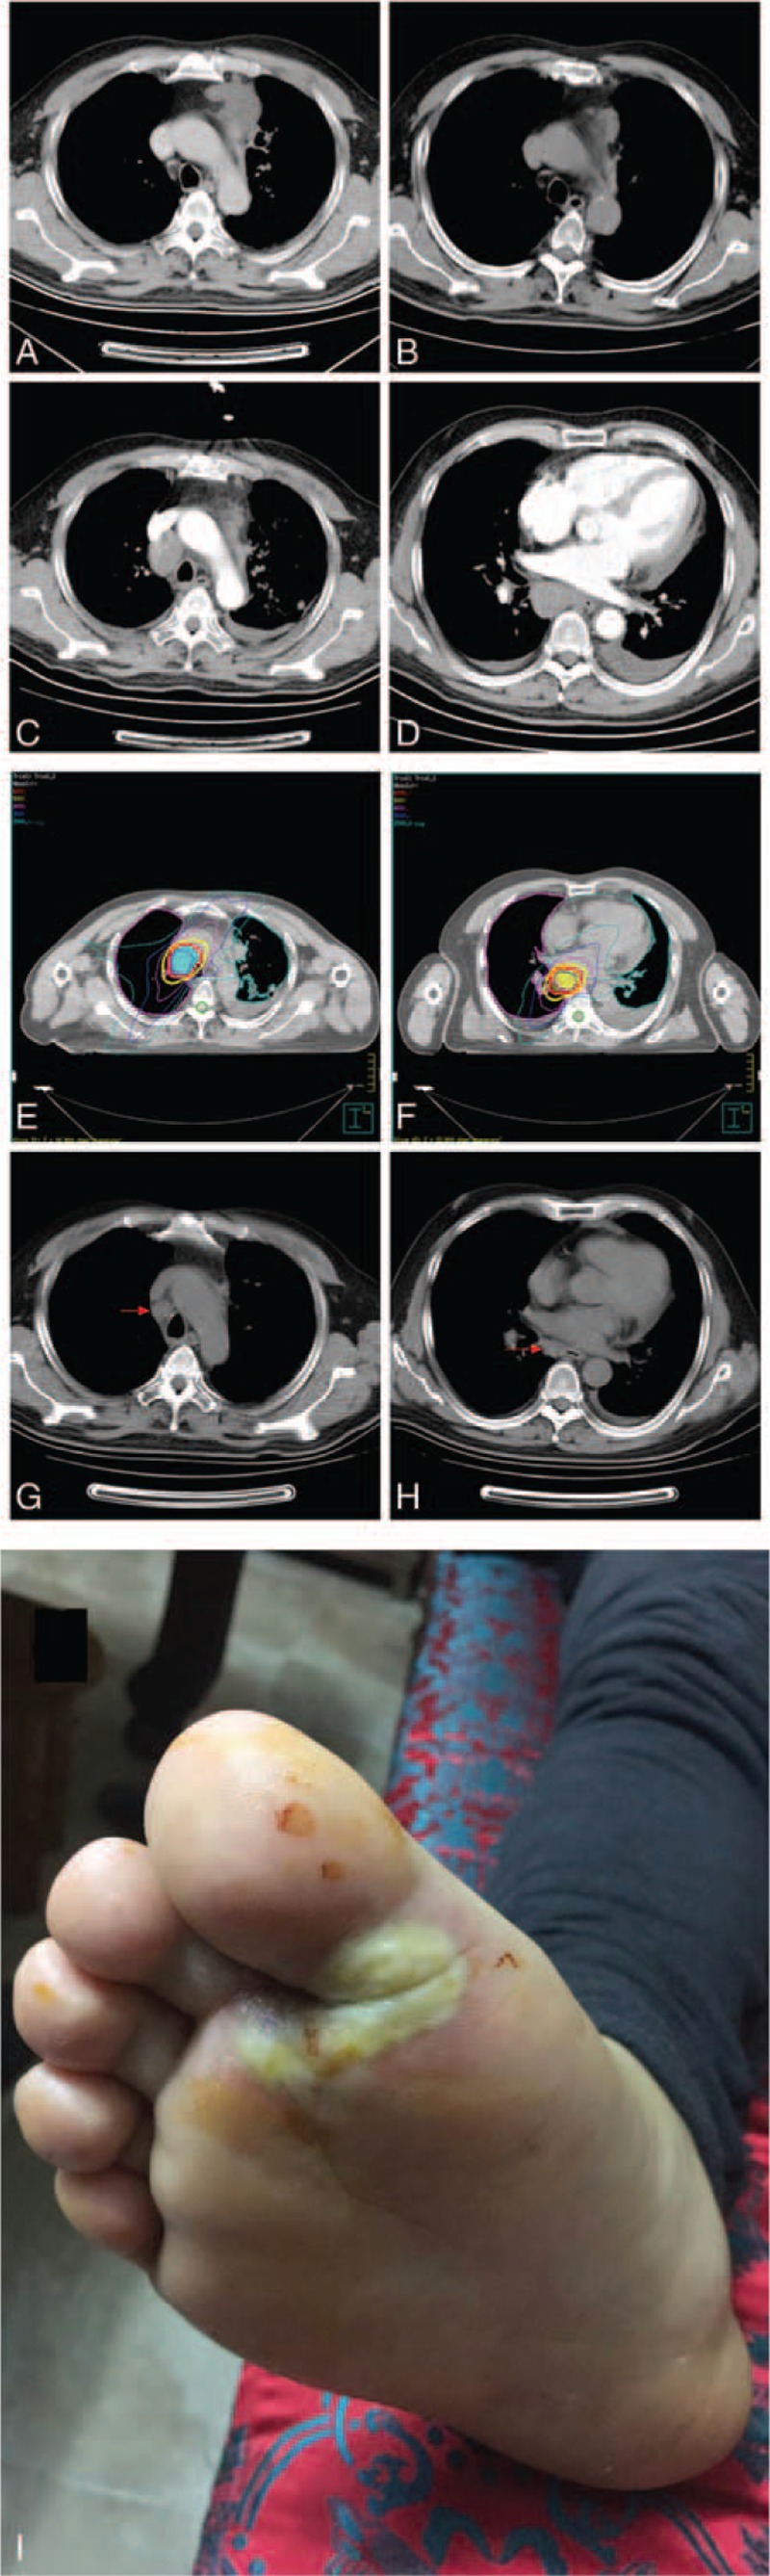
A: CT scan showed that there was a mass on left upper lobe (September 10, 2015); B: follow up CT scan showed that the mass on left upper lobe reduction; C and D: CT scan showed that there was lymph nodes metastasis in mediastinum (3A prevascular and right pulmonary ligament). Pleural effusion occurred at the thoracic cavity. Left upper lobe residual mass was steady; E and F: Radiotherapy (IMRT 2 Gy/fraction total DT: 40 Gy/20 fraction); G and H: One months later, CT scan showed that metastases lymph notes of mediastinum (3A prevascular and right pulmonary ligament) reduced partially, therapeutic evaluation partial remission (PR) and the mass of Left upper lobe residual reduced partially as well and pleural effusion reduced; I: The common side effects of apatinib was hand-foot syndrome. CT = computed tomography.
